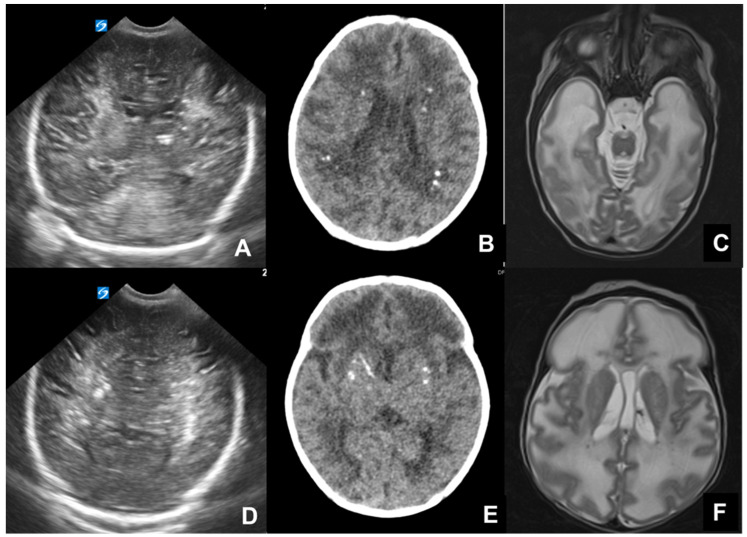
Twenty-day-old neonate affected by Aicardi-Goutieres type 1 syndromes, namely a pseudo-TORCH disease, showing white matter alterations, calcifications, and cystic vacuolization on US (A,D), CT (B,E), and MRI (C,F).
